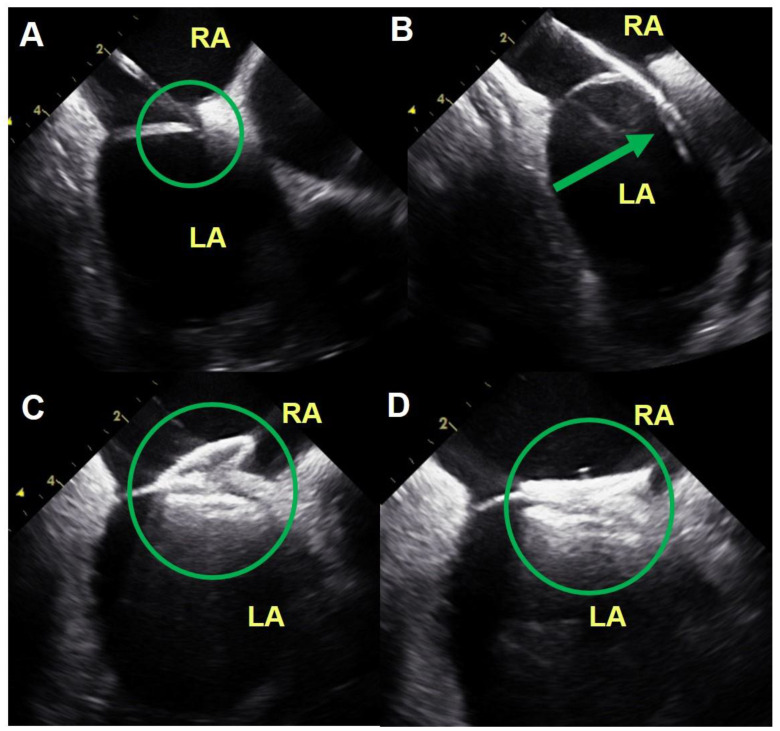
ICE-guided PFO device closure. The images were from a 68-year-old man who underwent successful PFO device closure without any complication ICE provides good images of the PFO inlet being pushed by a guiding catheter (A), passage of the catheter through the PFO (B), deployment of the PFO closure device (C), and good positioning of the device (D). ICE, intracardiac echocardiography; PFO, patent foramen ovale; RA, right atrium; LA, left atrium.
